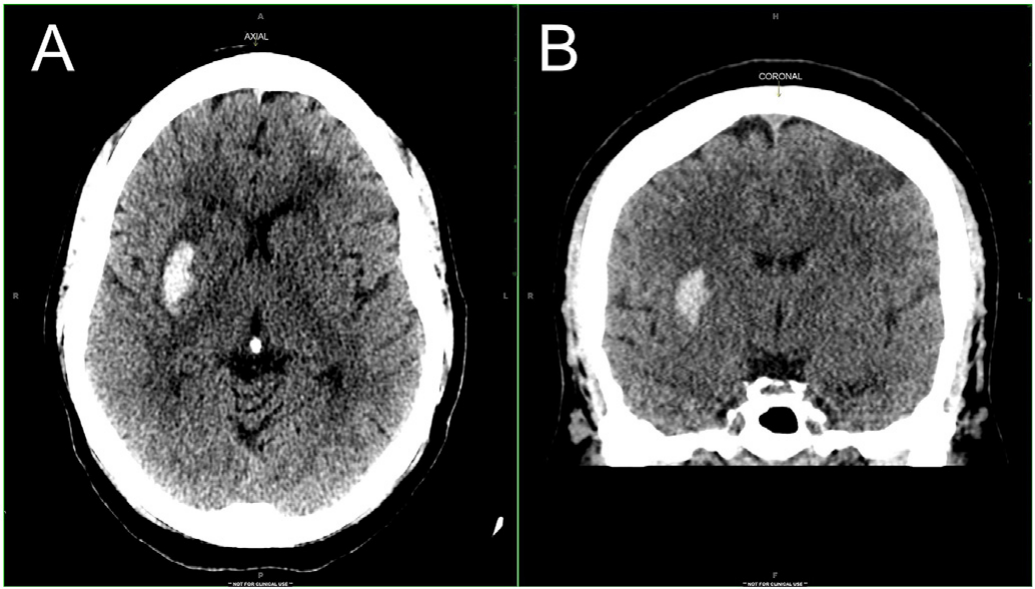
CT scan showing a right basal ganglion hemorrhage. (A) is an axial CT image that shows an ovoid shaped hyperdensity in the right putamen with minimal surrounding hypodensity, consistent with acute bleed surrounded by peripheral edema. (B) is a coronal CT image that shows an ovoid shaped hyperdensity in the right putamen, similar to the axial image. The images are diagnostic of a right-sided basal ganglia hemorrhage. CT, computed tomography.
